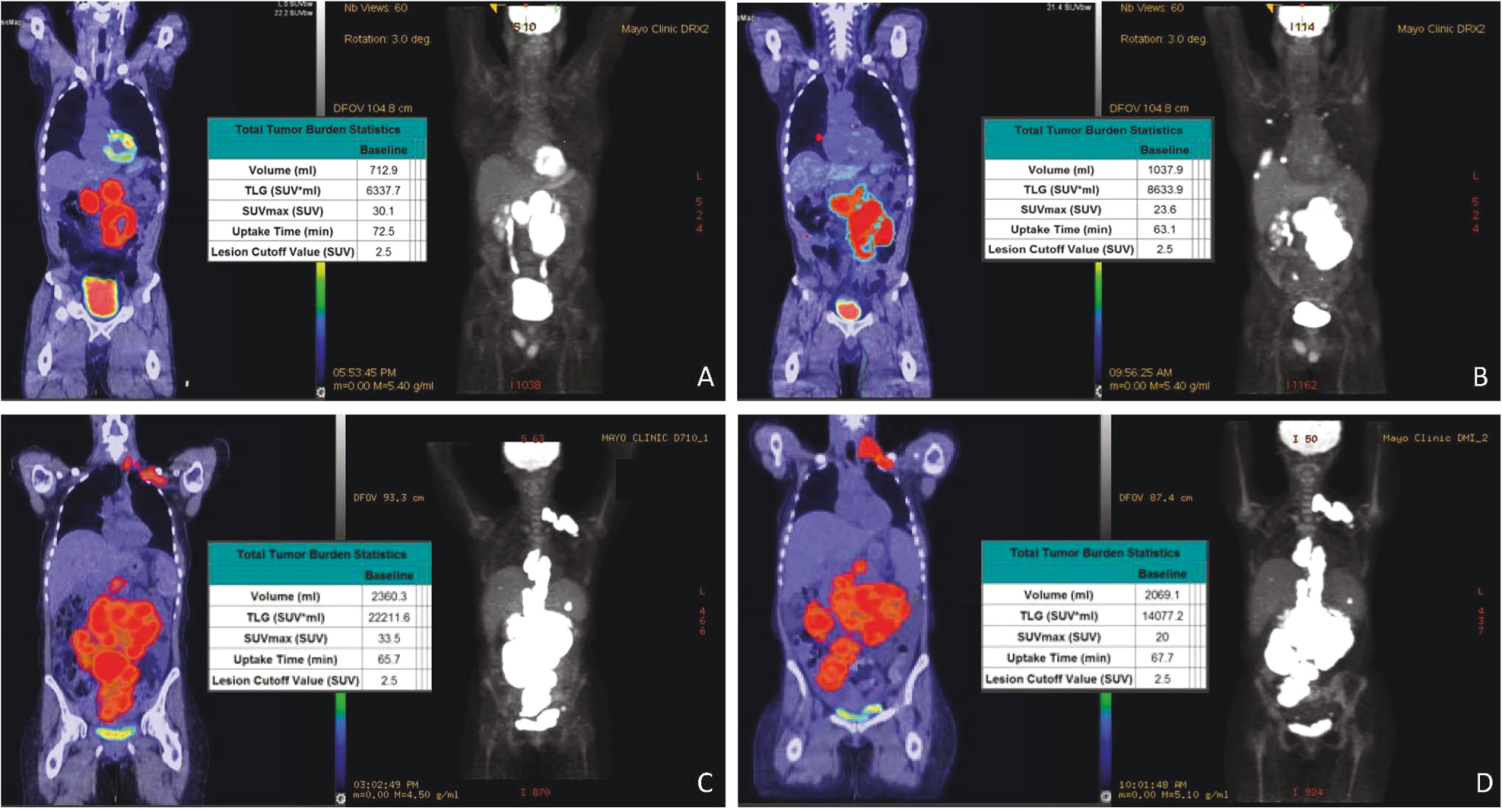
Representative Cases Demonstrating Metabolic FDG PET/CT Analysis. Pre-leukapheresis (pre-leuk) and pre- lymphodepletion chemotherapy (pre-LD) coronal fused PET/CT (left) and maximum projection image (right) PET/CT imaging and total tumor volumetric analysis for two patients. The first patient A, B had both increasing extranodal metabolic tumor volume (MTV) and increasing total lesion glycolysis (TLG) of the largest lesion (risk score 2) from pre-leuk A to pre-LD B, and experienced PD and death after CAR-T infusion. The second patient C, D had neither risk factor (risk score 0), is alive and without progression at last follow-up, despite having higher MTV and TLG at each individual time point compared to the first patient.
